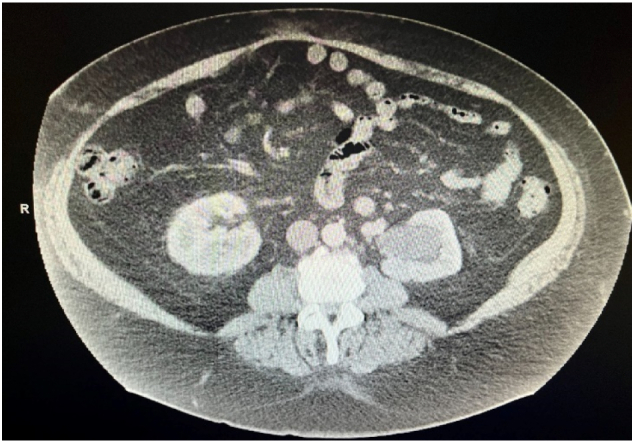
Axial image CT scan demonstrating left hydroureteronephrosis.
